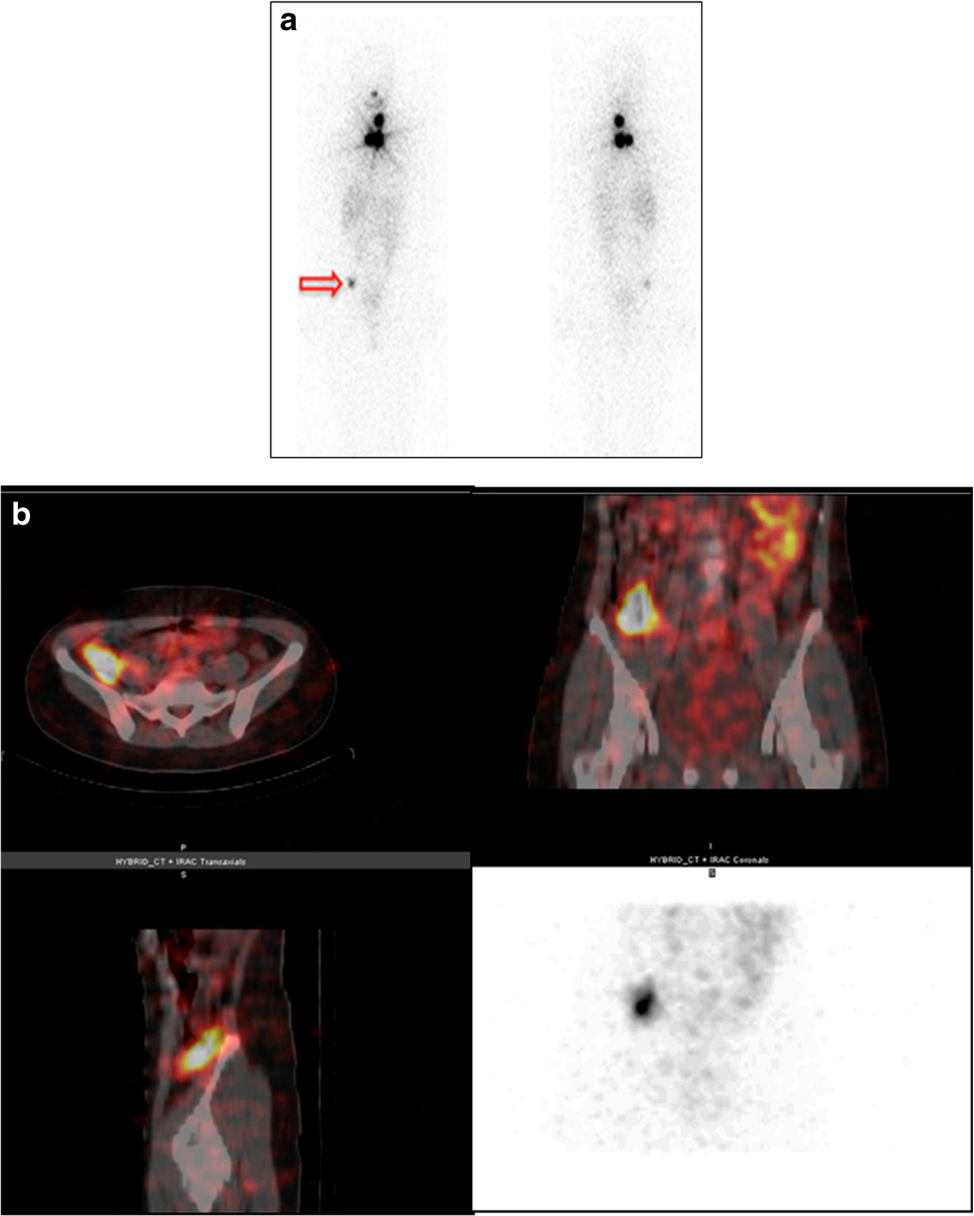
Post-ablation ^131^I-iodide SPECT/CT in differentiated thyroid cancer (DTC). A 48-year-old woman after thyroidectomy and lymphadenectomy for DTC (18 mm, infiltrating the thyroid parenchyma and capsule), with intravascular tumour emboli and nodal metastases found at surgery, stage pT1bN1bMx. The patient received 2.96 GBq ^131^I-iodide treatment. Planar post-ablation ^131^I-iodide scan (a) shows multiple sites of intense uptake in the neck and upper mediastinum consistent with known metastatic spread. There is an additional area of focal tracer accumulation of moderate intensity in the right pelvis (arrow). SPECT/low-dose CT slices and maximum intensity projection (MIP) image of the pelvis (b) locate this uptake to the lower portion of the ascending colon, consistent with physiologic tracer excretion. SPECT/CT excluded the presence of an additional malignant site in an equivocal finding detected on planar scintigraphy. *[Images provided courtesy of Drs. Paola A. Erba and Roberta Zanca, Regional Center of Nuclear Medicine, University of Pisa, Italy]*
